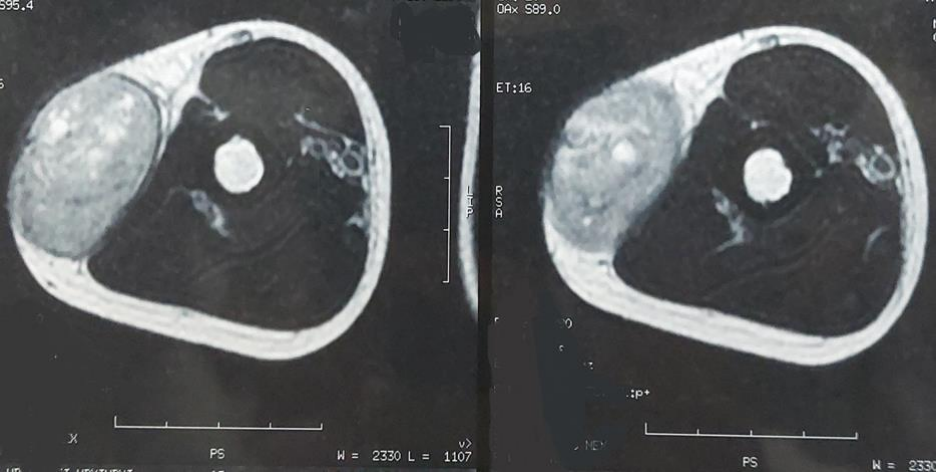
MRI of the right upper arm showing a supra-aponeurotic mass, heterogeneous in appearance, with blurred outlines, in contact with the aponeurosis of the triceps brachii muscle, without bone involvement
